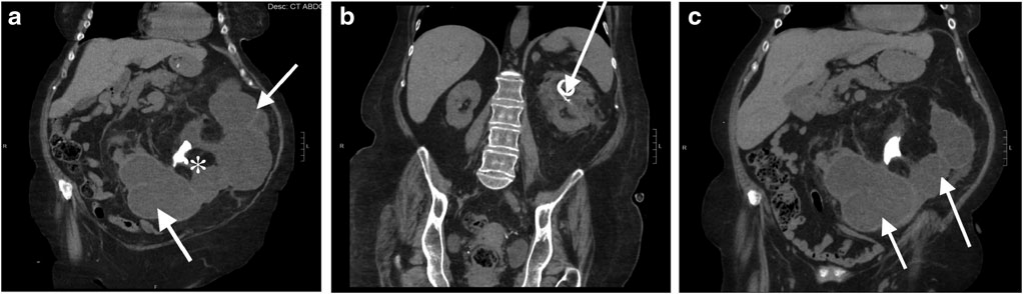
Noncontrast CT images of a 64-year-old woman with chronically obstructed horseshoe kidney. (a) Noncontrast CT (May 2016) shows left moiety of horseshoe kidney obstructed with staghorn calculus (*), causing massive pyonephrosis (*arrows*). (b) Marked interval improvement in superior pole pyonephrosis of left moiety of horseshoe kidney status postpercutaneous nephrostomy tube placement (*arrow*). (c) Persistent severe pyonephrosis (*arrows*) in interpole and inferior pole of left horseshoe kidney moiety prompted placement of second percutaneous nephrostomy tube.
